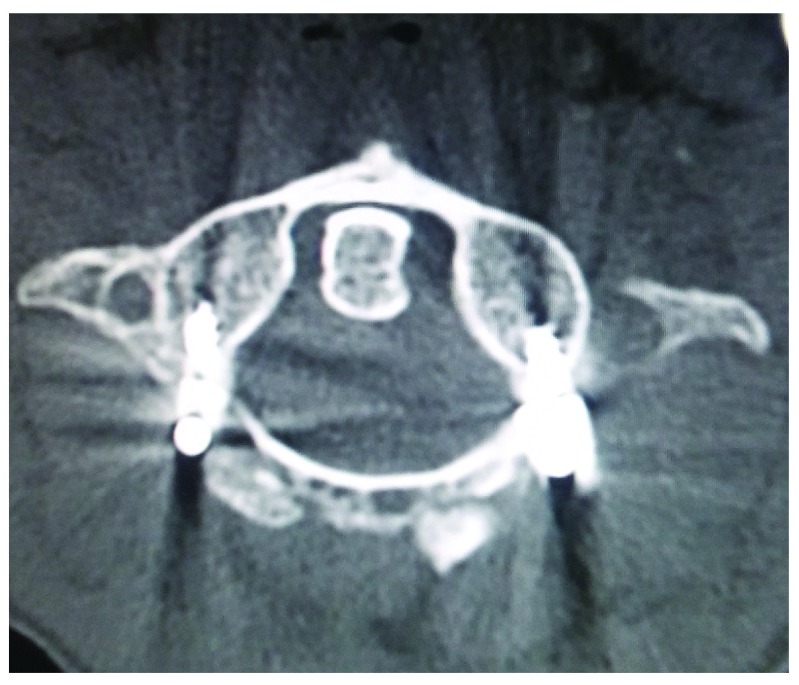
CT spine showing projection of screws through lateral mass of C1.
